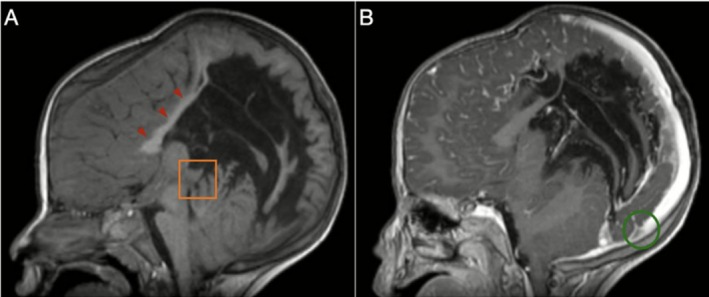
(A) Pre‐ and (B) post‐contrast sagittal T1‐weighted MRI images of the brain demonstrate dysgenesis of the corpus callosum (red arrowheads), predominantly involving its posterior segment, beaking of the tectal plate (orange square), and a low‐lying torcula (green circle).
